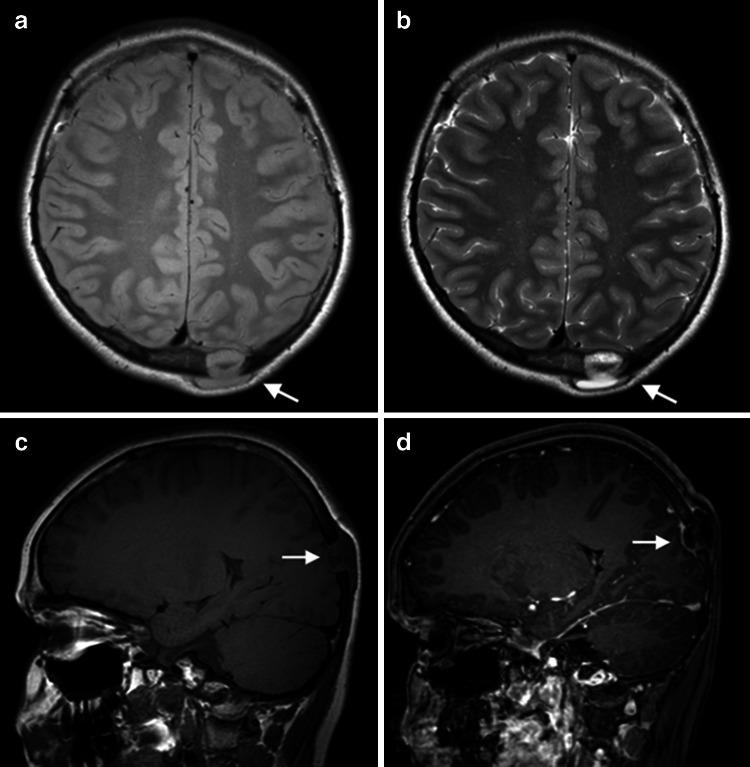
14-year-old male with Langerhans cell histiocytosis (LCH) presenting with a painless swelling on the skull. Axial proton density weighted image (a), T2-weighted image (b), and sagittal T1-weighted images before (c) and after the administration of intravenous contrast material (d). The lesion shows almost homogeneous T2 hyperintensity and slightly heterogeneous T1 intermediate signal intensity (*arrow* in a–c). After contrast material administration there is a predominantly peripheral enhancement pattern, less often seen in LCH lesions (*arrow* in d)
